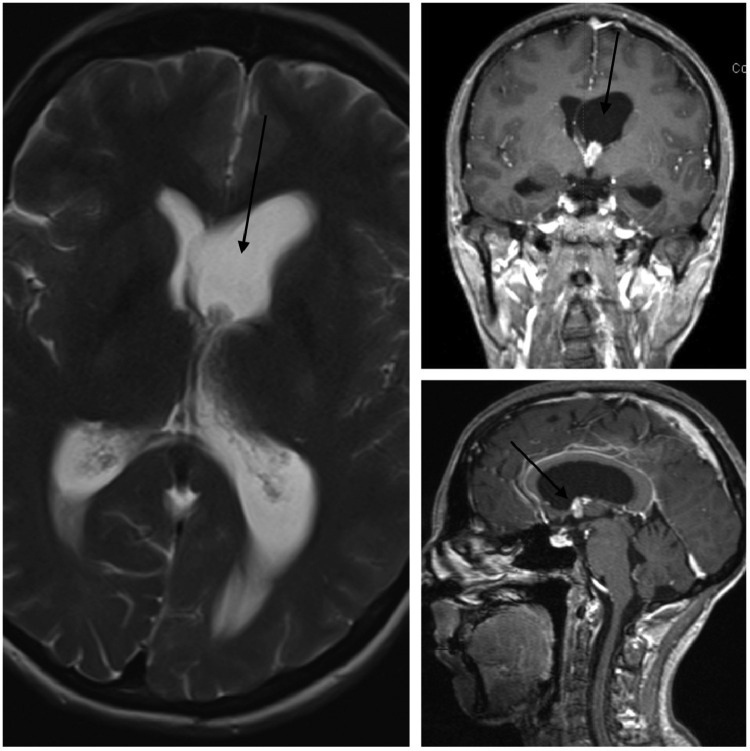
Pre-operative brain MRI with contrast exhibiting the presence of an avidly enhancing lesion at the left foramen of Monro protruding to the third ventricle roof with a lobulated, band-like enhancement adjacent to the lesion within the inferior portion of the left lateral ventricle (resembling continuation of the lesion).
